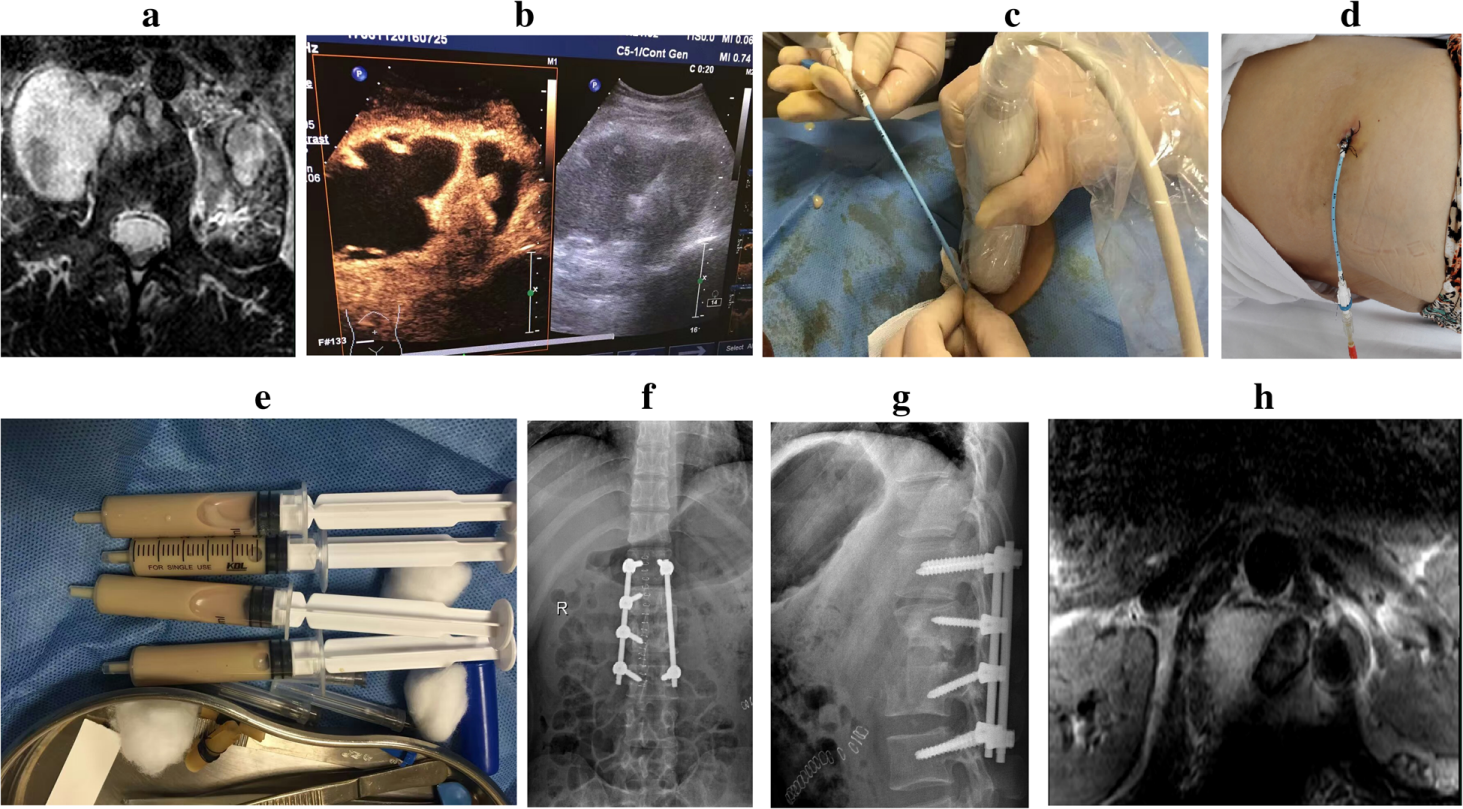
A 46-year-old female patient with tuberculosis of the L1/2 lumbar vertebrae and PA underwent preoperative PCD under ultrasound guidance, antituberculosis therapy for 3 weeks, posterior pedicle screw fixation, and anterior debridement and bone graft fusion. a. Preoperative magnetic resonance imaging (MRI) showing lumbar spinal tuberculosis, with abscess formation. b–e. PCD was performed under ultrasound guidance. f, g. The X-ray image 3 days after surgery showed good internal fixation and bone grafting. h. Postoperative MRI performed at the 3-month follow-up showed complete debridement and no obvious abscess
